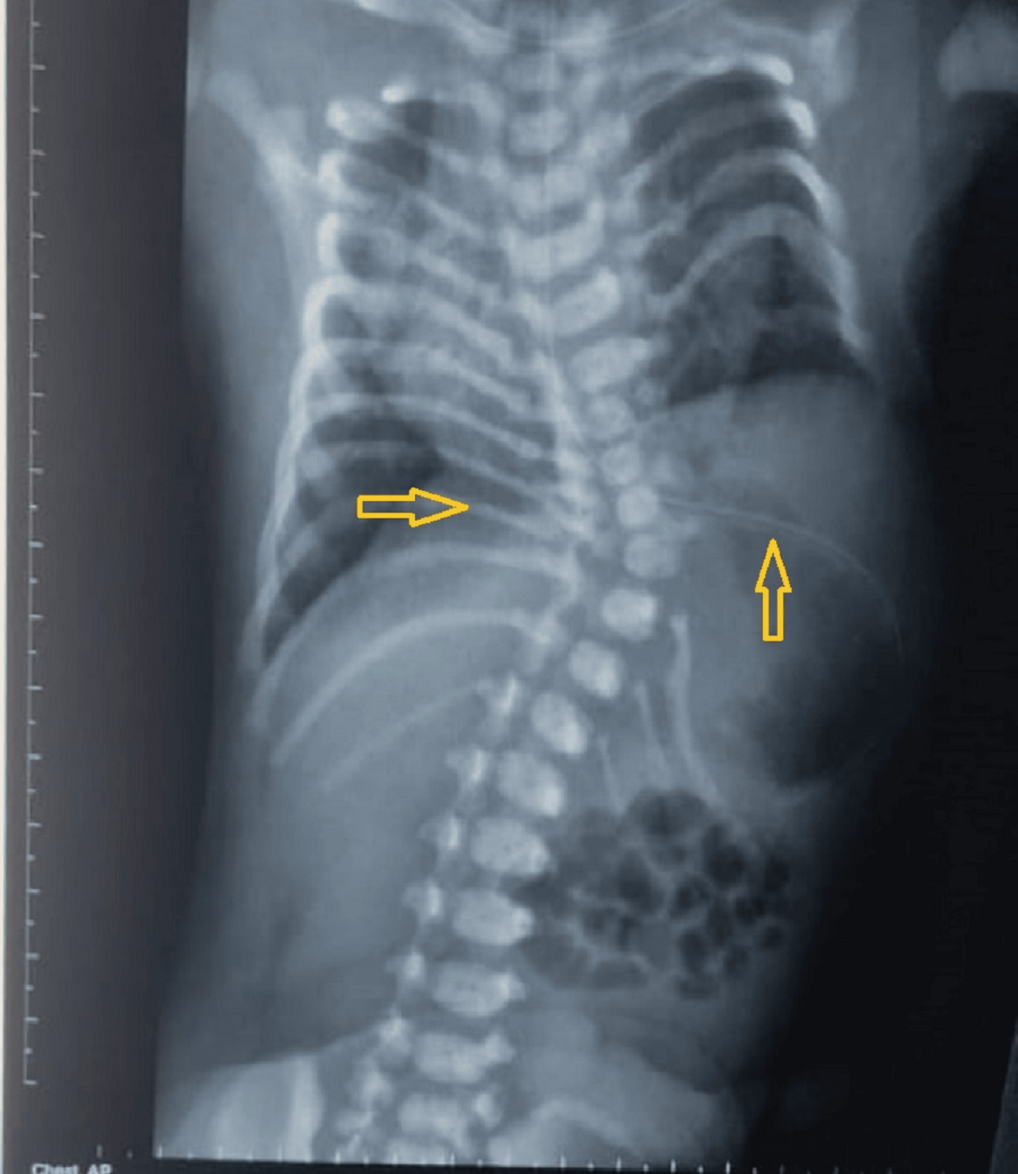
X-ray chest AP view of a neonate with spondylo-thoracic dysplasia. Shows scoliosis, displacement of left lower ribs, and fusion of the spondylo-costal joint, crab deformity. This pathognomonic feature arises from posterior fusion and anterior flaring of the ribs, giving rise to the typical crab-rib deformity [3]. AP: Antero-posterior.
